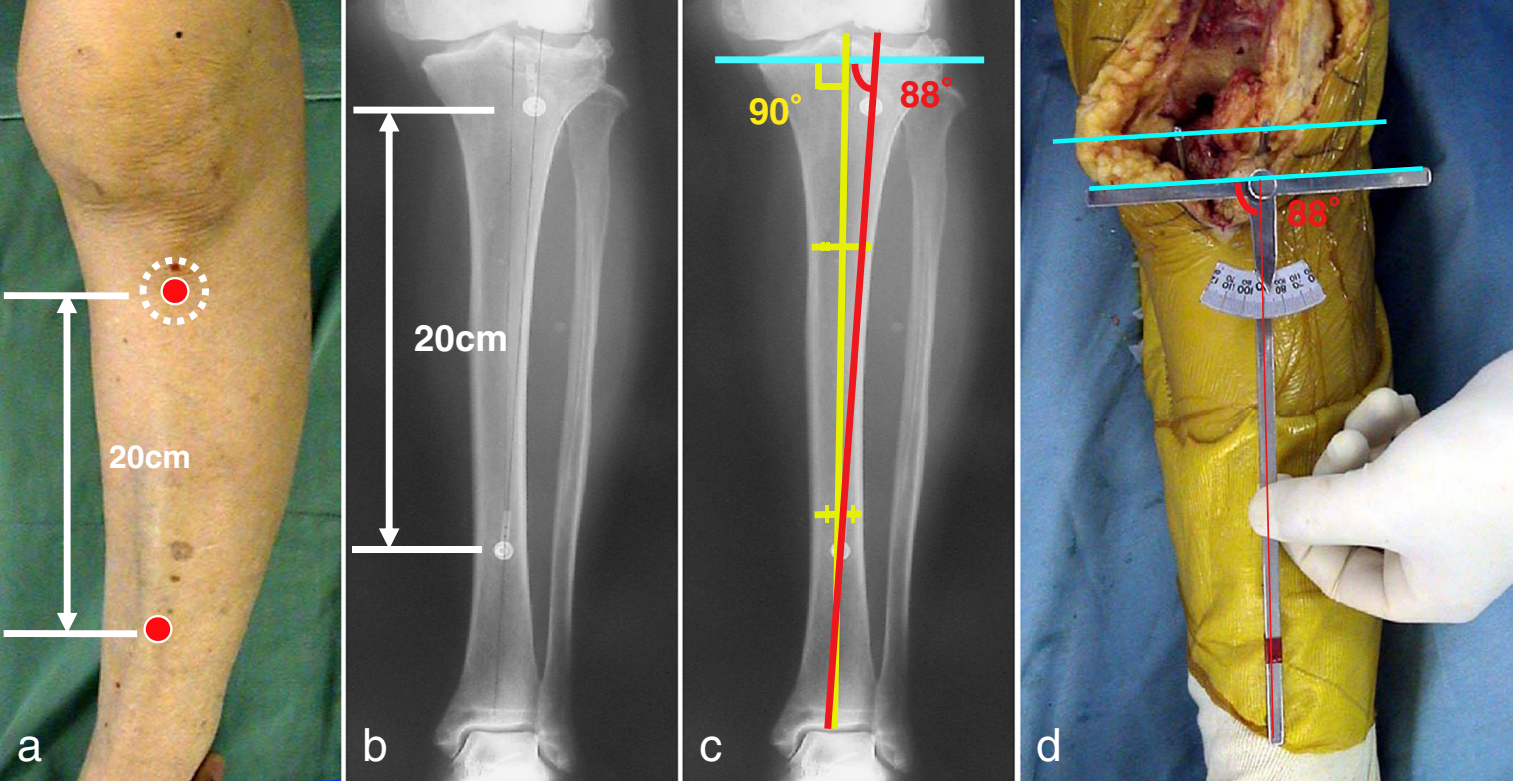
Preoperative planning (a–c) and intraoperative protractor method (d). (a) Two metallic markers are placed on the skin, one at the tibial tuberosity and the other at the anterior tibial crest. (b) The markers are 20 cm apart. Anteroposterior radiographs of the leg are obtained. (c) We defined the line going along the two markers as the tuberosity line (*red line*). The osteotomy line (*blue line*) is perpendicular to the anatomical axis of tibia (*yellow line*). We calculated the angle between the tuberosity line and the anatomical axis of tibia. Finally, we calculated the angle of the osteotomy line with the tuberosity line and called it the osteotomy angle. In this case, the angle of the osteotomy line with the tuberosity line is 88°. (d) The 20-cm bar is in accord with the tuberosity line, and the 10-cm bar is in synchrony with the osteotomy line. We set the osteotomy angle of the protractor based on a preoperative measurement. In this case, the protractor is set at 88°. We cut the bone parallel to the osteotomy line (*blue line*) determined by the protractor.
